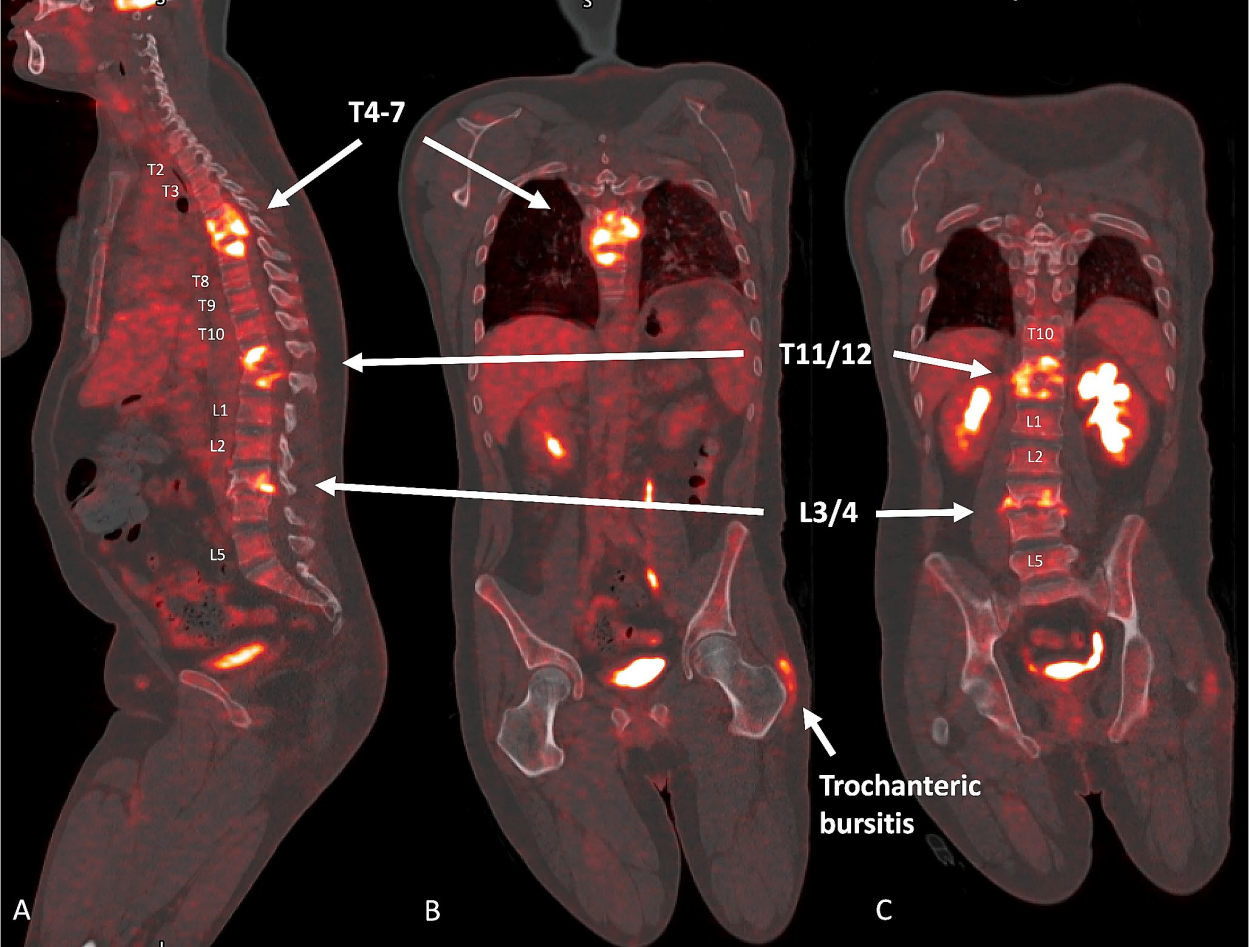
PET/CT before treatment initiation A: Whole-body 18-FDG-PET/CT sagittal view. B: Whole-body 18-FDG-PET/CT coronal view thoracic spine. C: Whole-body 18-FDG-PET/CT coronal view thoracolumbar spine
